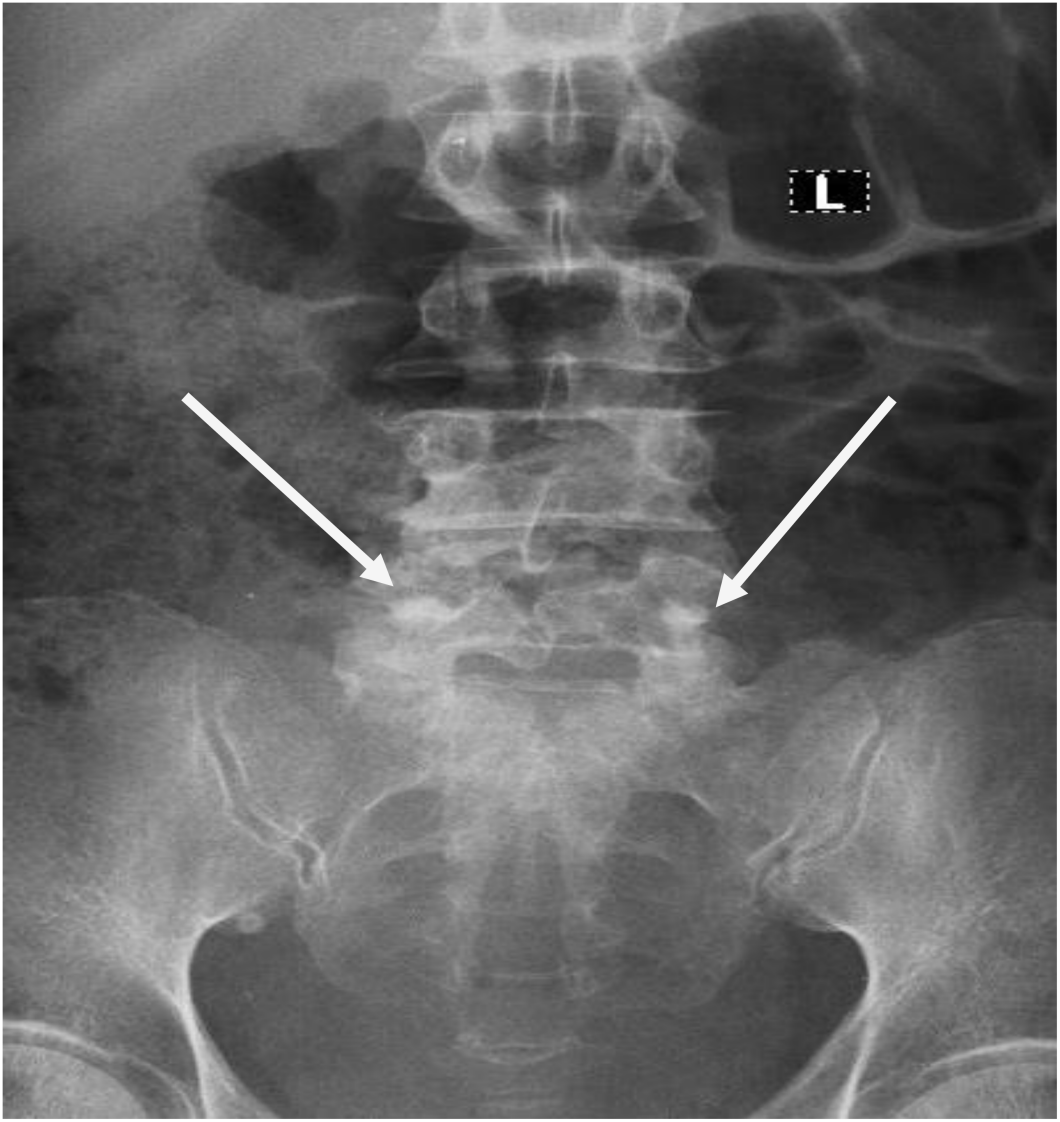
Lumbosacral Spine X-rays; AP-view. Shows suspiscious osteoblastic lesions on L5 (white arrows).
